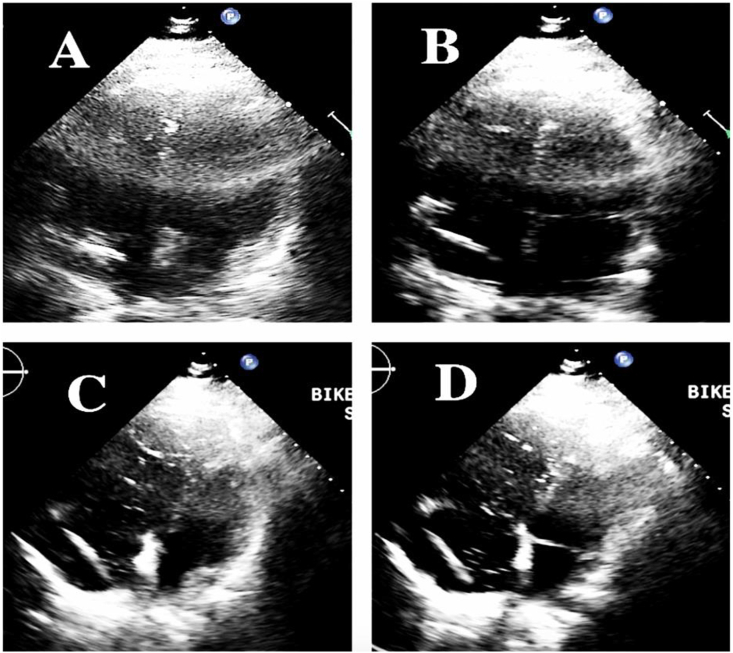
Leftward ventricular septal shift during exercise. Panels A and B show baseline echocardiographic four chamber views in diastole and systole, respectively. Panels C and D are the same views during exercise, highlighting RV dilation and a reduction in LV volume.
